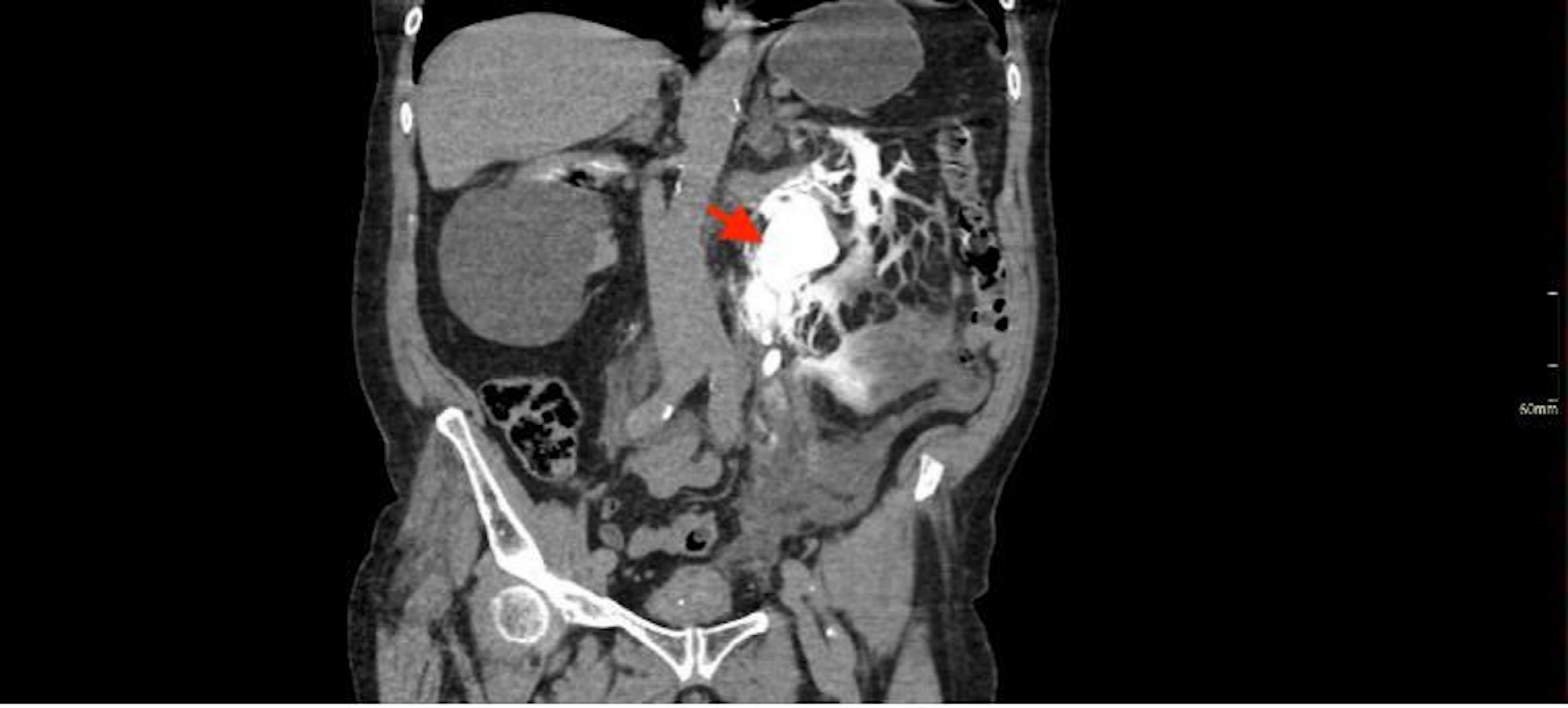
CT Urogram—Coronal section; delayed phase image demonstrating contrast extravasation from the left renal calyx and hold up of contrast in the left excretory system
